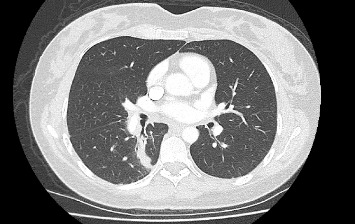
CT chest 3 months after treatment with alectinib, showing a significant reduction in the size of the right lower lobe lung lesion.
